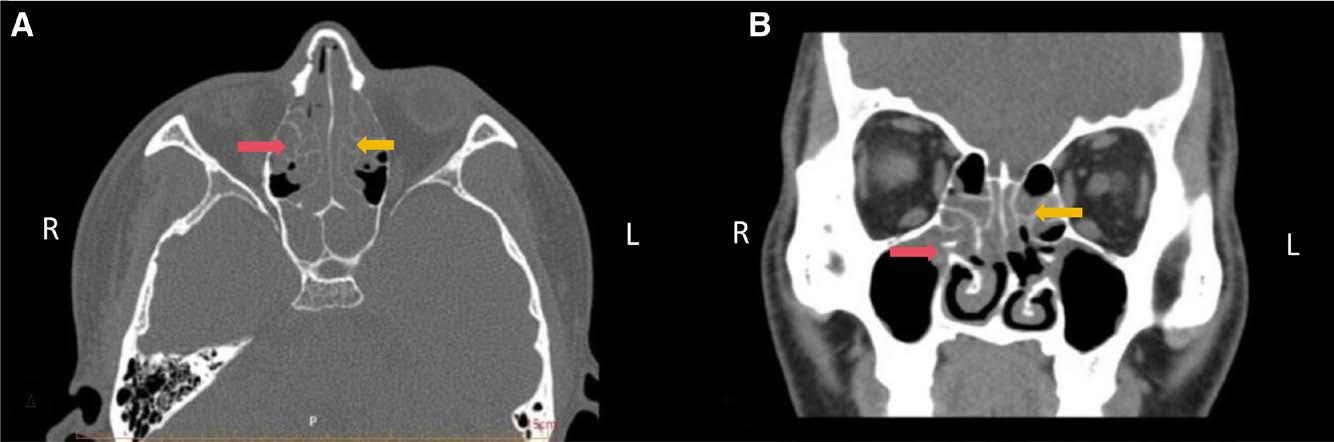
(A) Axial CT of the nasal cavity. (B) Coronal CT of the nasal cavity. Yellow scissors indicate a nasal polyp in the low-density area of the left nasal cavity; red scissors indicate REAH in the left nasal cavity with low density. CT = computed tomography, REAH = respiratory epithelial adenomatoid hamartomas.
